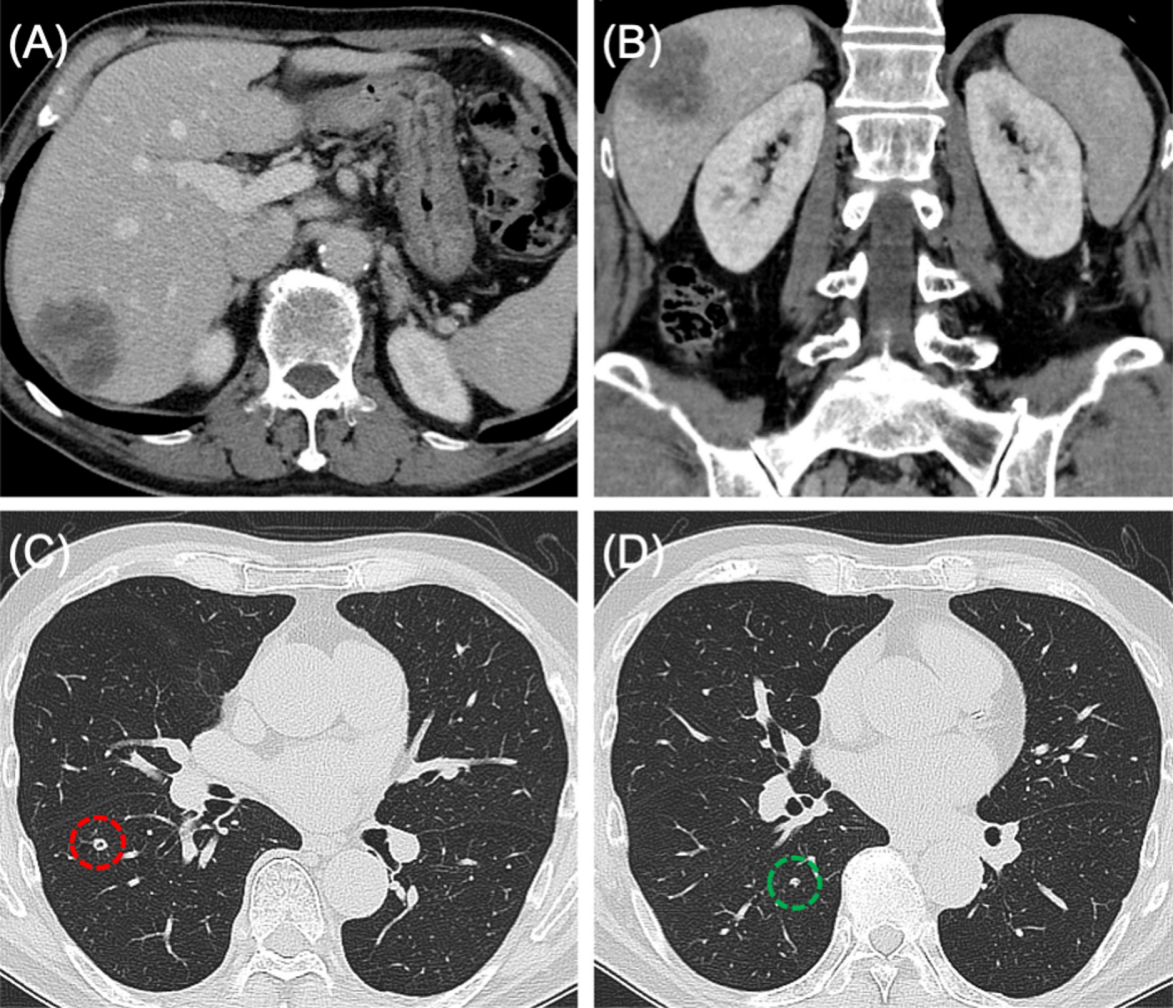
Initial diagnostic images of the metastatic tumors before treatment. Computed tomography demonstrating a solitary metastatic liver tumor (4.3 × 3.3 cm) in the posterosuperior segment (A, B) and two lung tumors in the right basal lobe (red and green dotted circles) (C, D).
